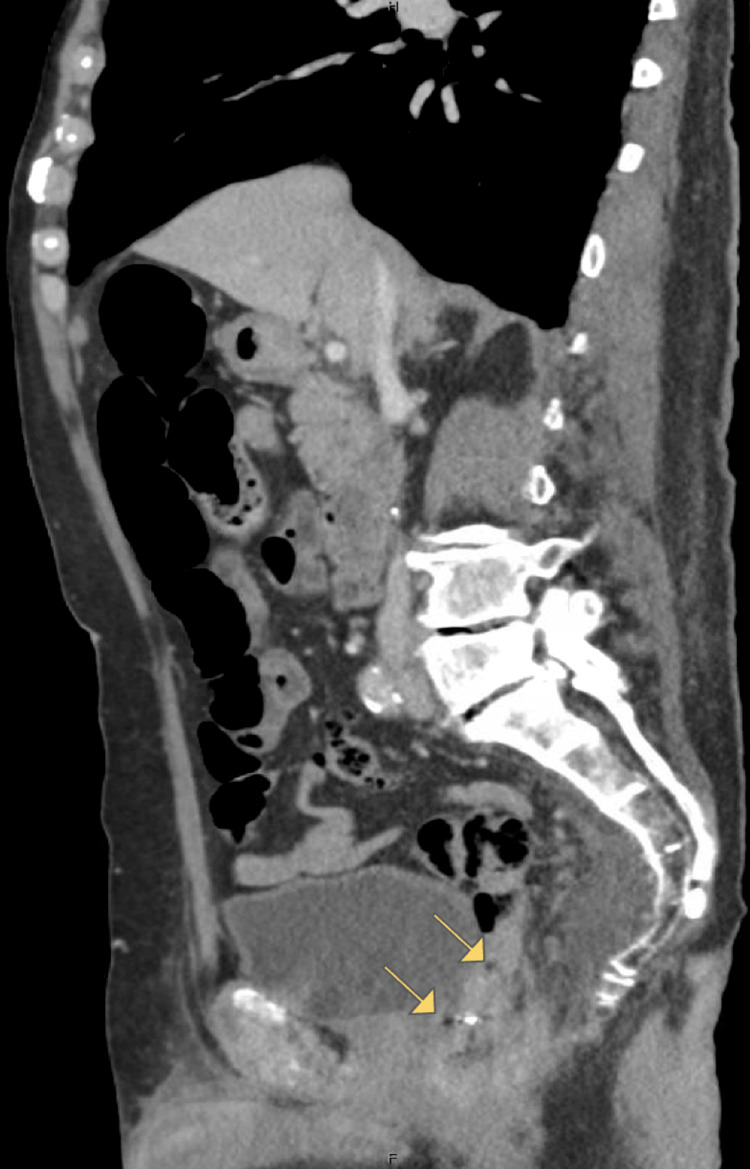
CT imaging showing heterogenous perianal fluid collection and segmental mural thickening of the rectum, as well as spots of intraperitoneal air (arrows) adjacent to the anastomotic staple line. CT = computed tomography
